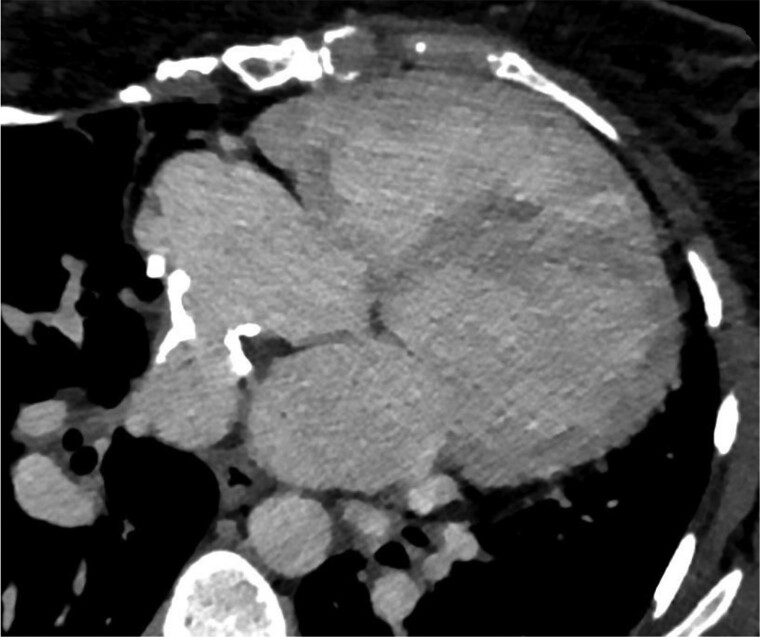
Computed tomography scan of stenotic pulmonary venous baffle.
